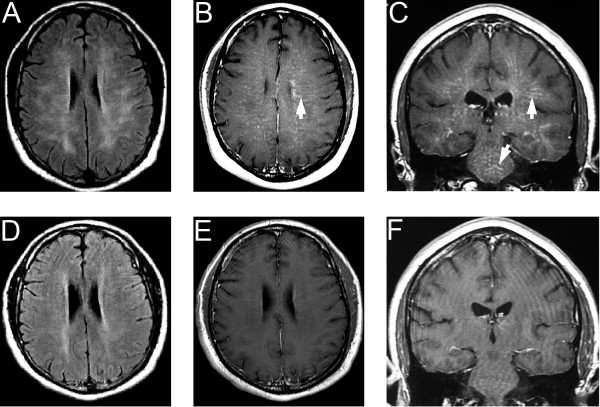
Brain magnetic resonance images before (A-C) and after (D-F) treatment. (A) T2-weighted FLAIR imaging demonstrates bilateral, diffuse hyperintense lesions in the white matter with sparing of the cortical U fibers. T1-weighted images following intravenous gadolinium administration in the (B) axial and (C) coronal planes show bilateral, symmetrical, linear contrast enhancement in a radial distribution throughout the white matter of the cortex and brainstem (arrows). (D) Following treatment with steroids and cyclophosphamide, T2-weighted FLAIR imaging demonstrates a dramatic improvement of the hyperintense abnormalities and almost complete resolution of gadolinium enhancement on the T1-weighted images in the (E) axial and (F) coronal planes.
